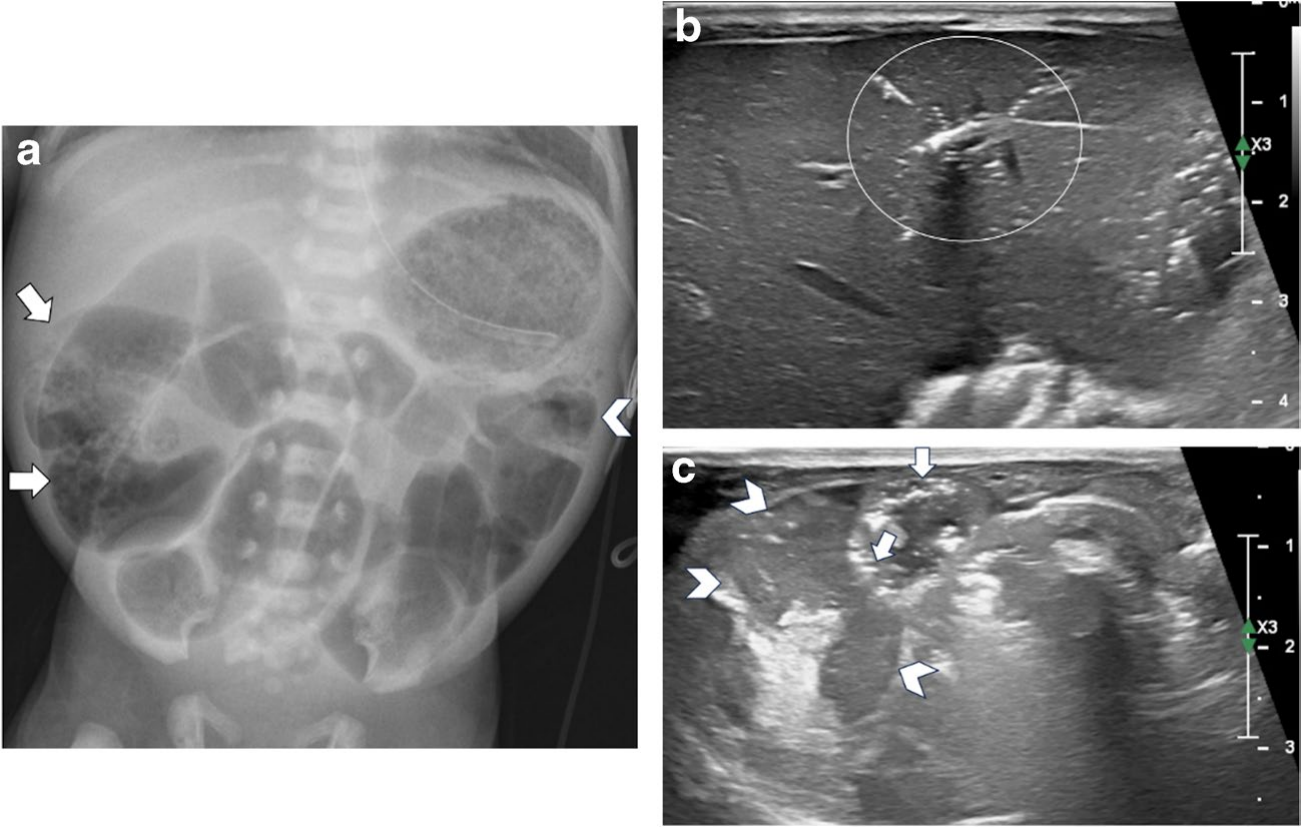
A 9-day-old female born at 29 4/7 weeks (case 10 from Table 4). Ultrasound imaging upgraded the modified Bell’s stage from 2 A to 3B. a An AP supine abdominal radiograph demonstrates gaseous distended bowel loops with pneumatosis on the right (*arrows*) and to a lesser degree, on the left (*arrowhead*). b Transverse midline abdominal ultrasound image performed 85 min later reveals a small amount of portal venous gas in the left hepatic lobe (*circle*). c Transverse right lower quadrant ultrasound image demonstrates pneumatosis (*arrows*) and complex, predominantly echogenic extraluminal fluid (*arrowheads*)
